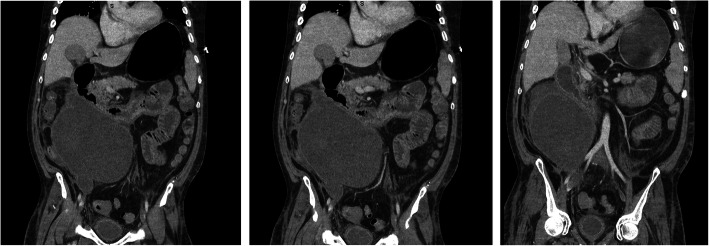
Coronal CT sections showing a large retroperitoneal haematoma, duodenal compression and gastric distension and a distorted left ventricle
